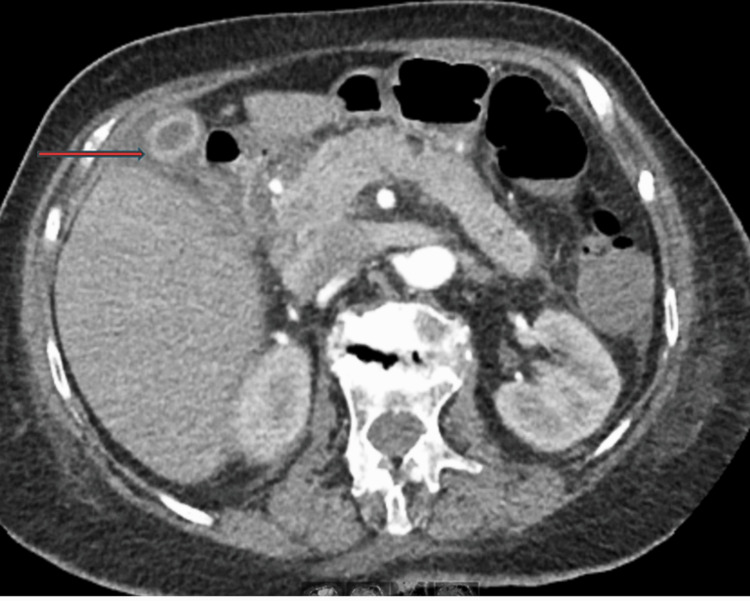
CT abdomen/pelvis with IV contrast (axial view) Prominent mural thickening/edema, measuring up to 1 cm in thickness circumferentially. Mild mucosal enhancement is seen as well as pericholecystic fluid, consistent with cholecystitis:  Red arrow
